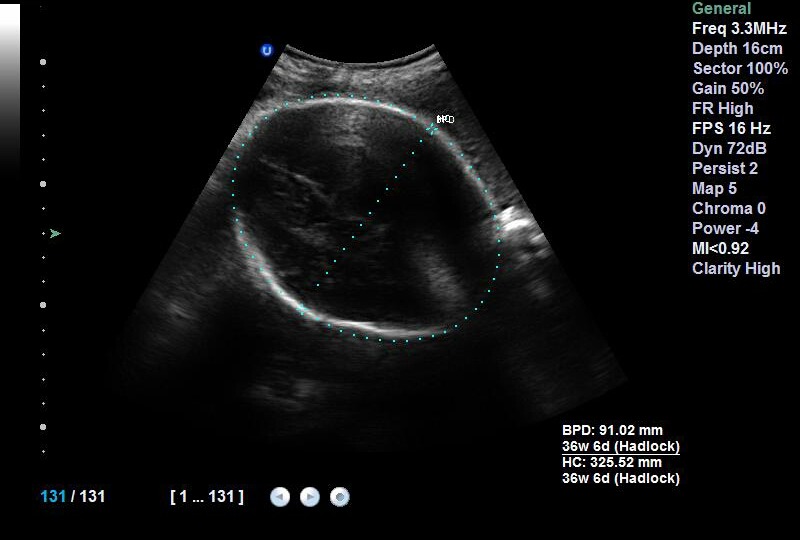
Sonographic image of myoma
